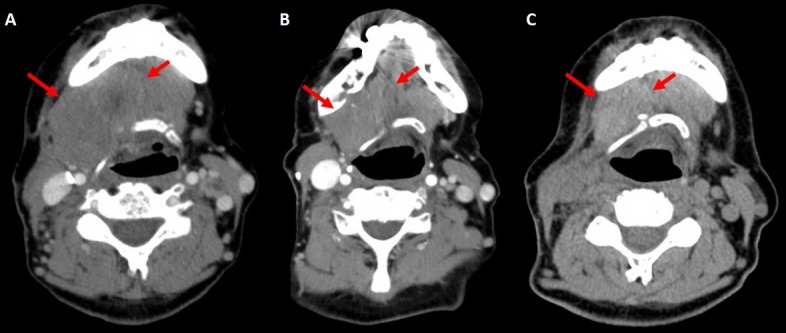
Local response to radiation (A) Computed tomography (CT) of the neck on October 28th, 2016. (B) Same performed on November 21st, 2016 after completion of three fractions of palliative irradiation, showing a reduction in the size of the main lesion as well as a reduction in size and numbers of adenopathy. (C) CT scan of the neck on March 28th, 2017. Unfortunately, IV-contrast was not used, so accurate assessment was difficult. However, the bulk of the disease had reduced in size.
